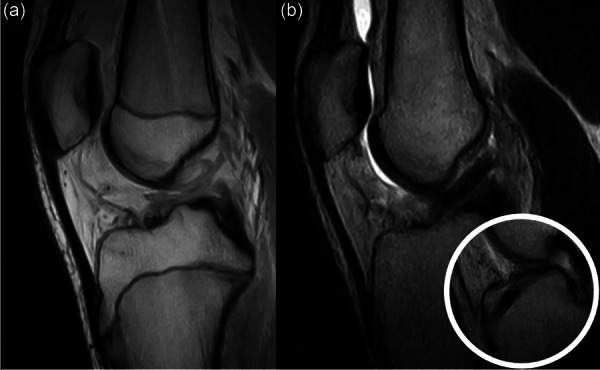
Preoperative magnetic resonance imaging (MRI) of a 14.2‐year‐old male footballer with anterior cruciate ligament (ACL) injury (a) and MRI at 2‐year follow‐up after physeal sparing over‐the‐top ACL reconstruction and Lateral Tendesis (b), showing good signal and inclination of the ACL graft.
